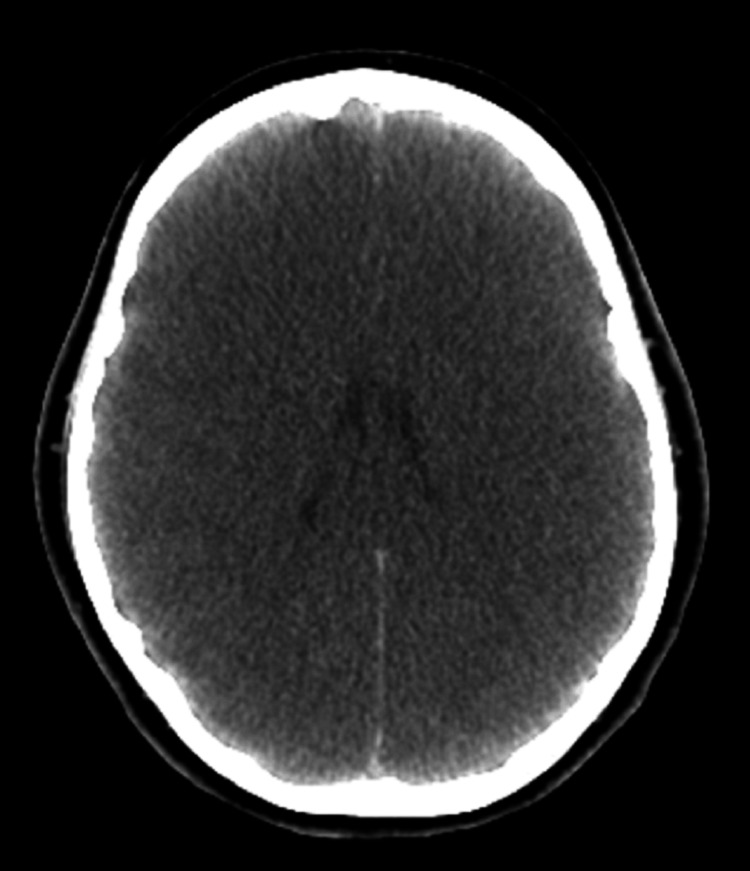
CT brain revealed evidence of cerebral herniation.
